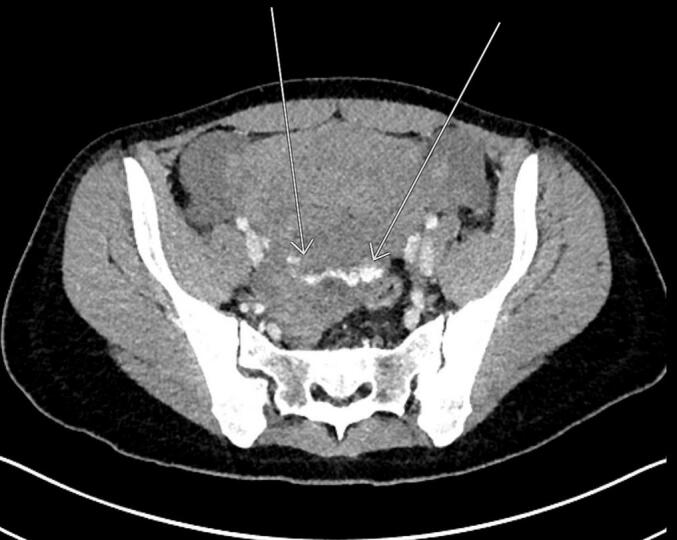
CT scan of the second patient shows diffuse bleeding from the uterus into the abdominal cavity.
